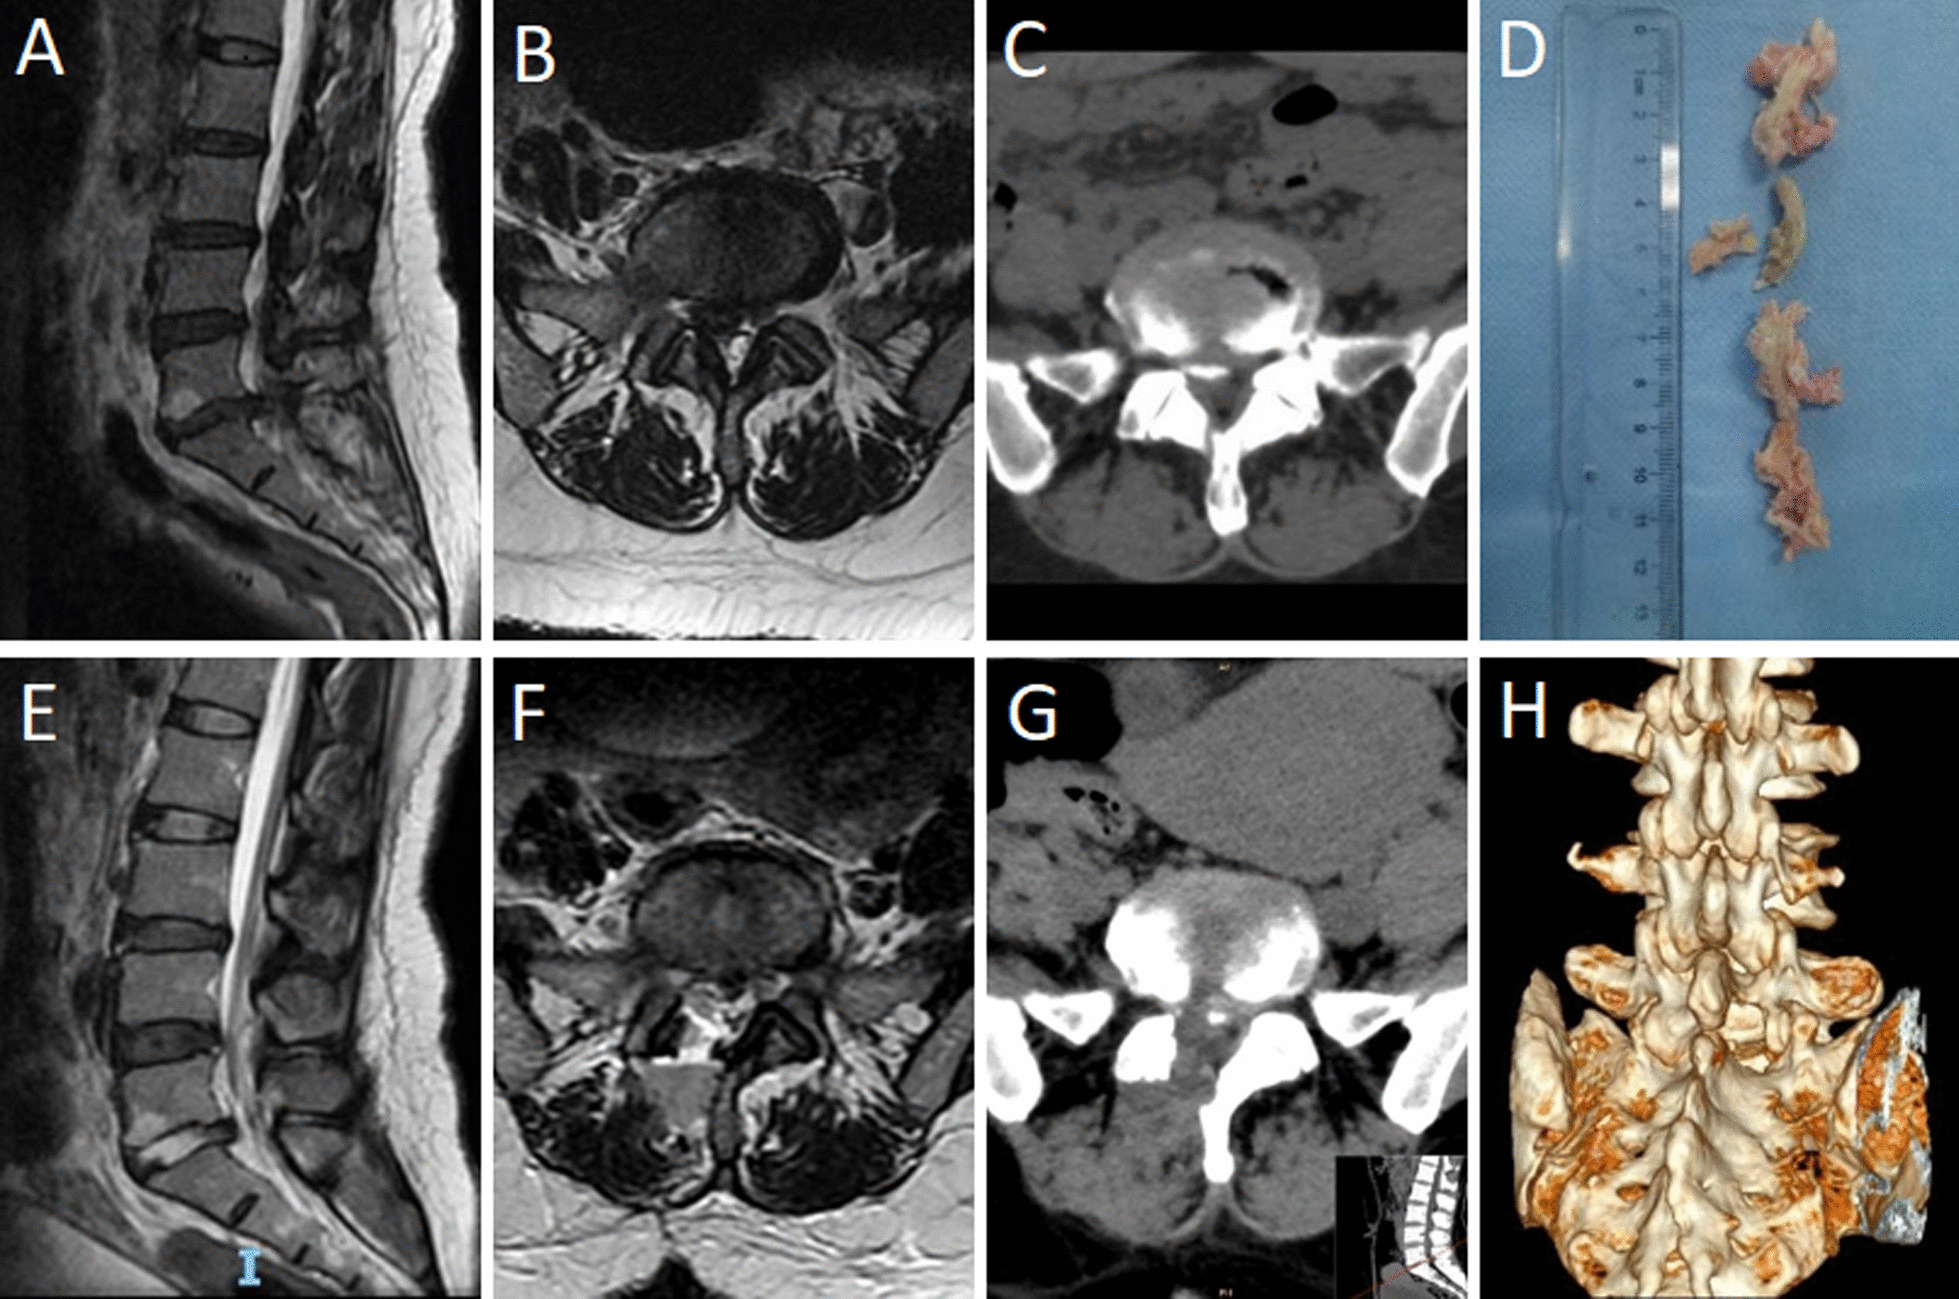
Patient, female, 50 years old, L5-S1 lumbar disk herniation, treated with OSE technology. A–C Preoperative MRI and CT showed L5-S1 intervertebral disk herniation and compression of the right S1 nerve root. D Removed nucleus pulposus tissue. E, F Postoperative MRI showed that nucleus pulposus tissue was removed, and the spinal canal area was significantly increased. G, H Postoperative 3D CT showed that the facet on the surgical side was preserved, the calcified tissue was partially resected, and the bone was limited resection
